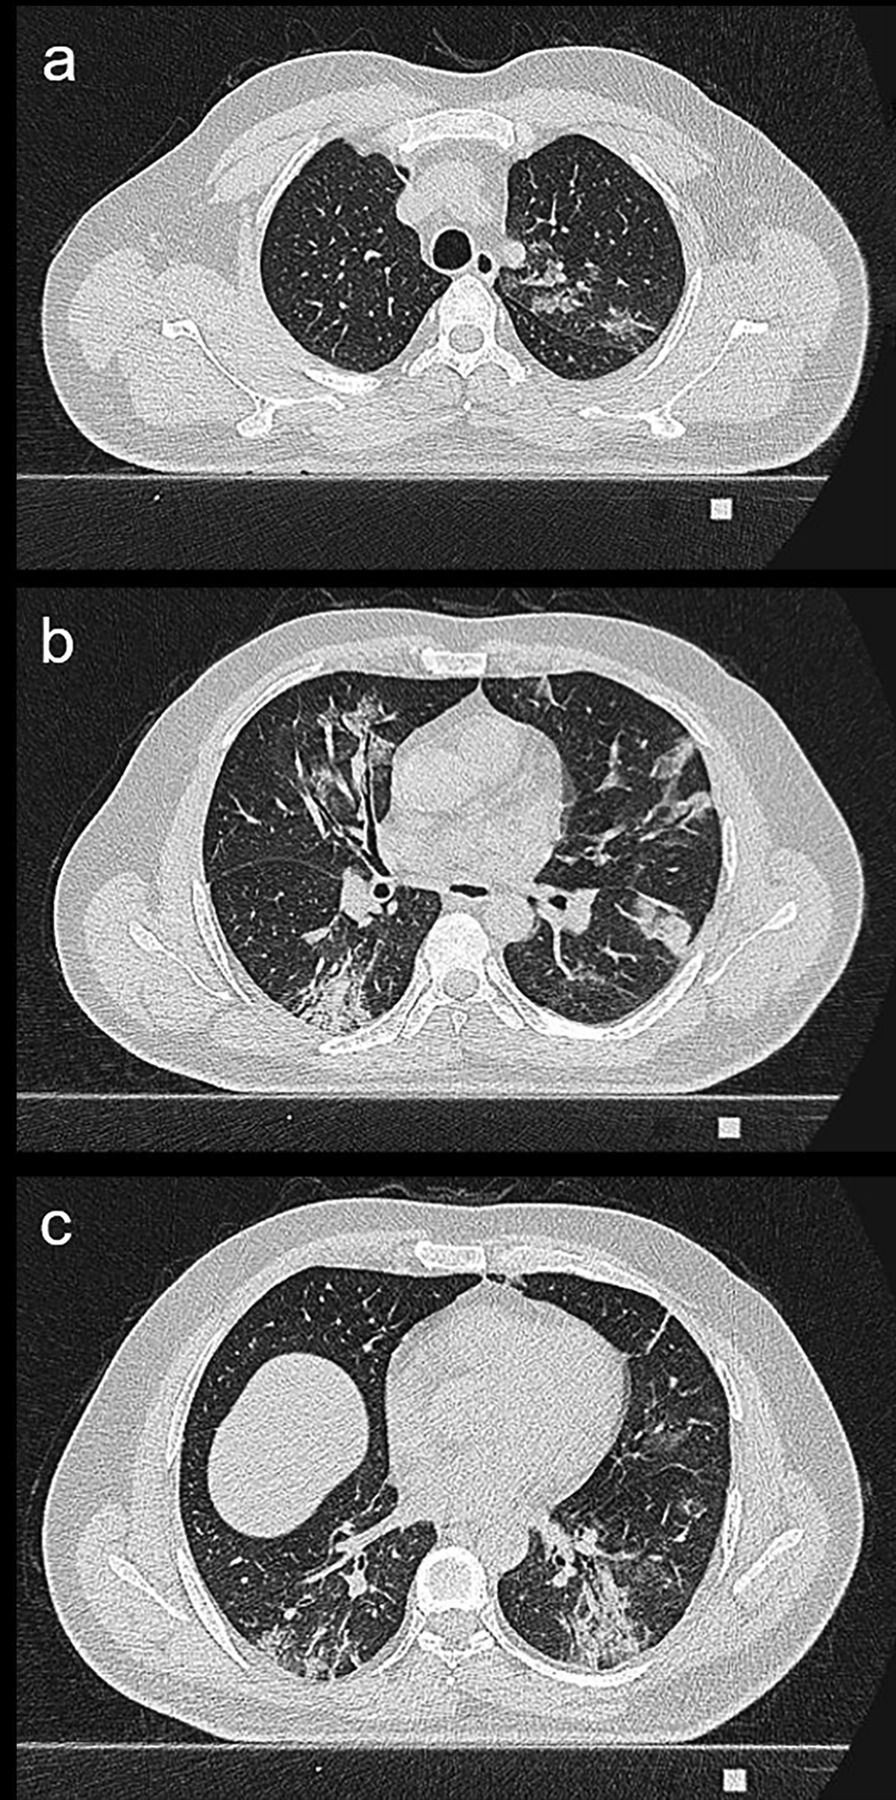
65 years old male patient. According to the CO-RADS category in terms of Covid pneumonia, a CO-RADS 5 patient had a mixed parenchyma pattern (pattern 2), and the patient's total CT score value was 11 and the structured total CT score value was 22 according to a, b and c
